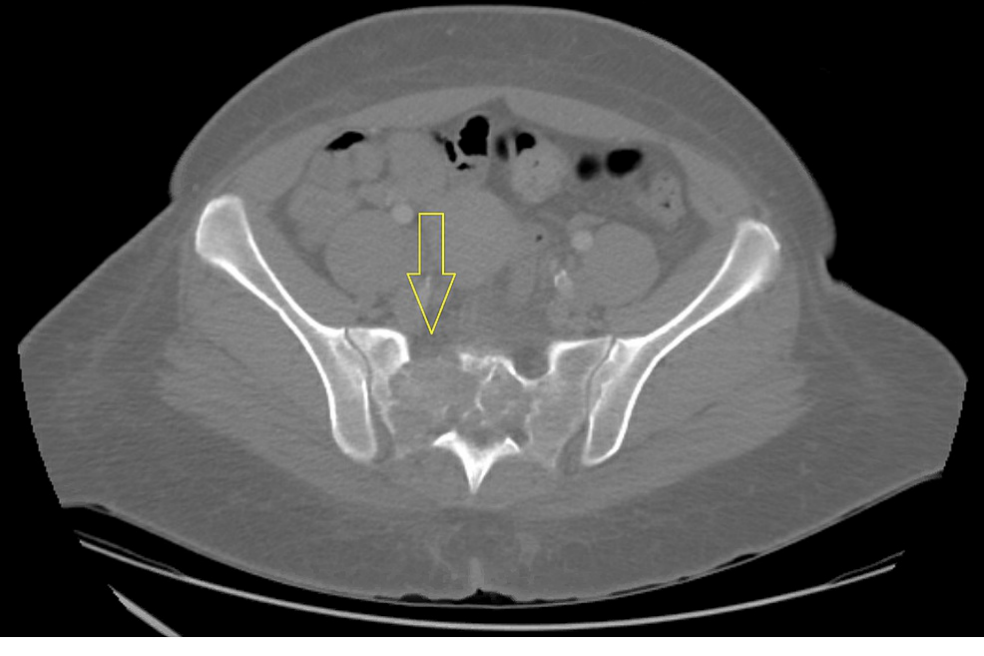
Computed tomography of the pelvis showing interval enlargement of the right sacral lytic mass crossing the midline and eroding anterior and posterior cortex of the sacrum.
